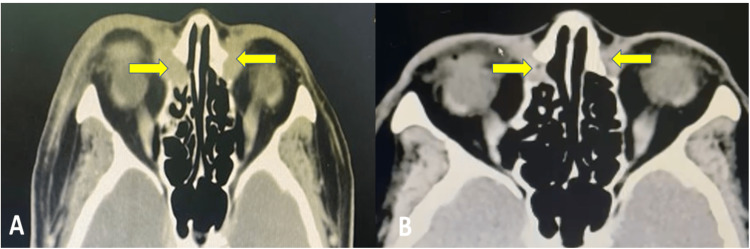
A) Pre-chemotherapy and B) Post-chemotherapy of CT scan (axial view) showing reduction in the size of homogenous soft tissue mass in lacrimal sac.
